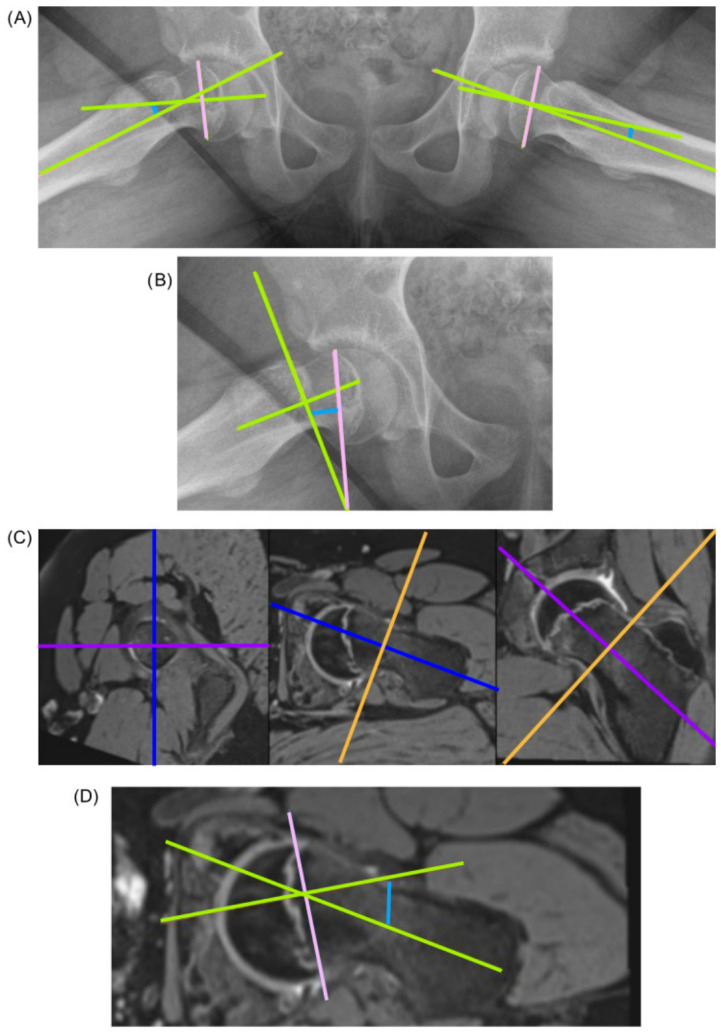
Primary outcome images. (A) Southwick angle evaluated using the radiographic frog-leg view; (B) posterior epiphyseal slip angle evaluated using the radiographic frog-leg view of the affected side; (C) maximum deformity plane definition using 3D MRI of the affected side; (D) posterior epiphyseal slip angle evaluated using MRI of the affected side.
